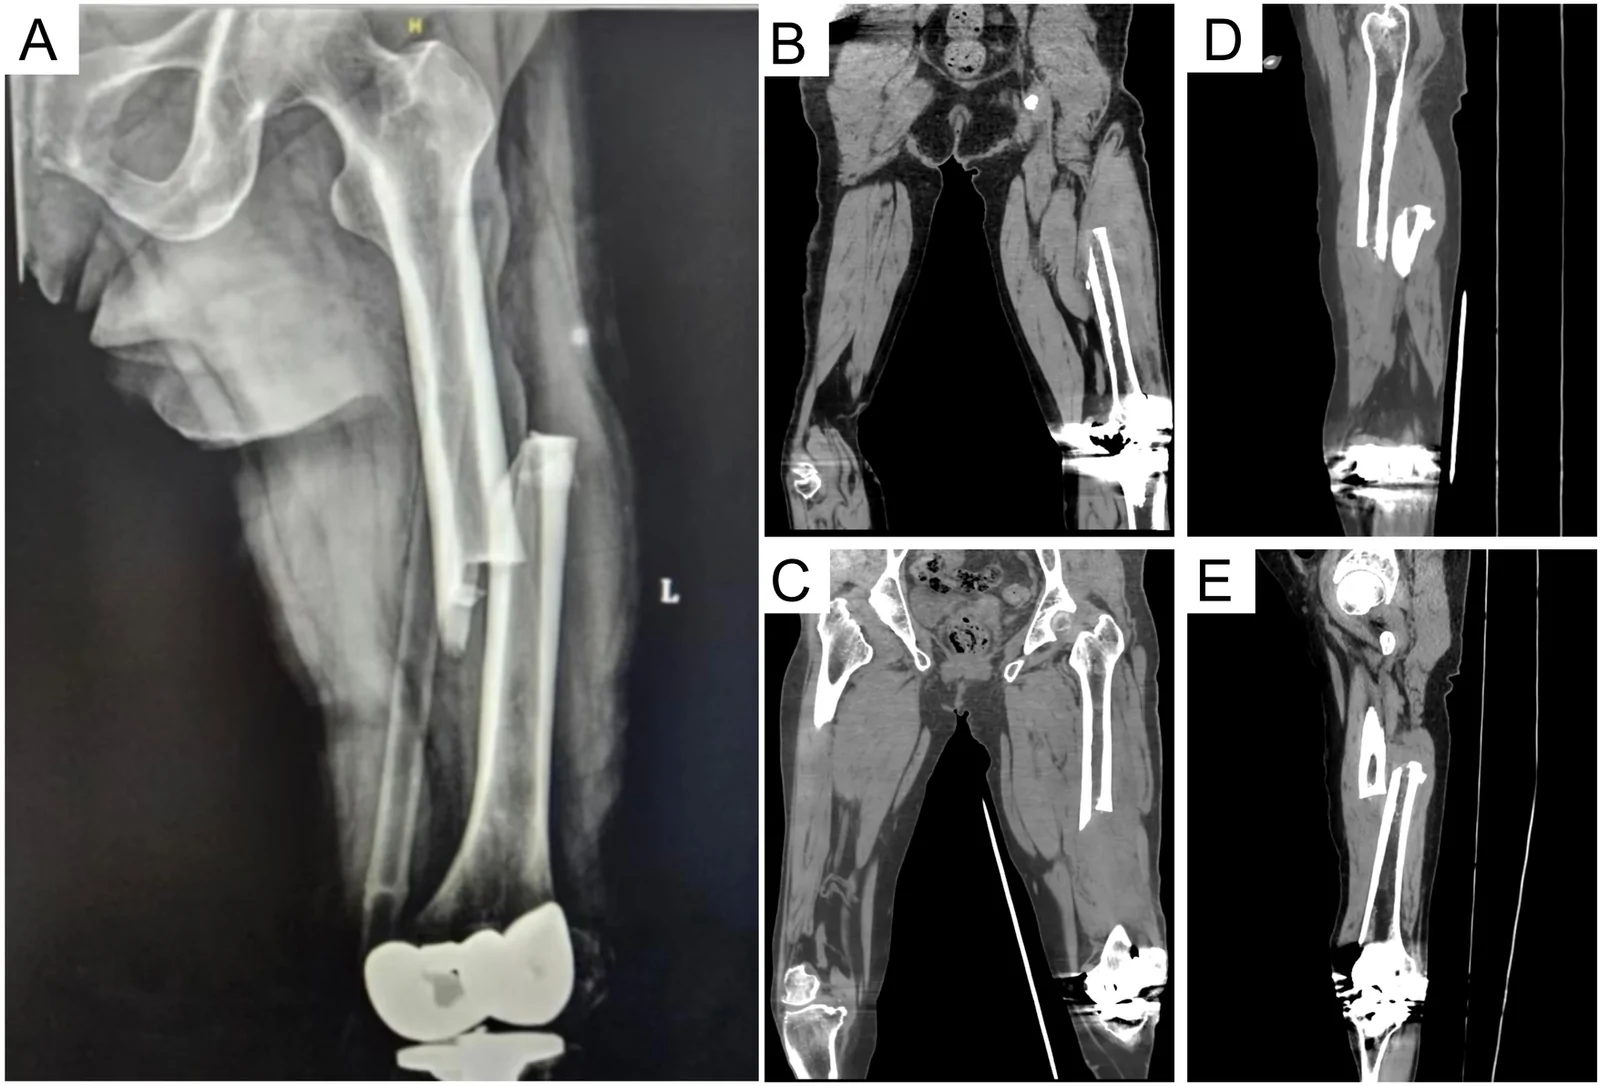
Preoperative imaging of the left femur. (A) Anteroposterior full-length radiograph of the left femur. (B,C) Coronal high-resolution CT images. (D,E) Sagittal high-resolution CT images.
